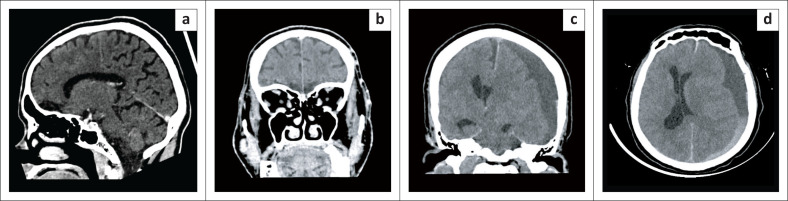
Trauma related pathology as a cause of adult-onset seizures. (a and b) Bilateral rectus gyri gliosis in a 49-year-old male with new-onset seizures. Sagittal and coronal contrast enhanced CT (CECT): Hypodensities of bilateral rectus gyri consistent with gliosis. (c and d) Left acute subdural hematoma in a 72-year-old male with new-onset seizures and confusion. Axial and coronal non-enhanced CT (NECT): Large left mixed density subdural hematoma with a haematocrit level. Associated subfalcine and uncal herniation with right lateral ventricle entrapment hydrocephalus.
